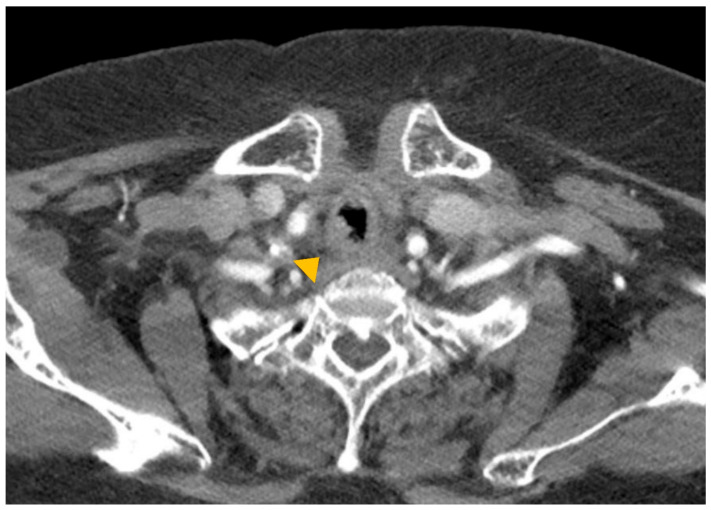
Neck computed tomography image. A diffuse wall thickening and intraluminal irregularity are noted in the proximal trachea (arrowhead).
